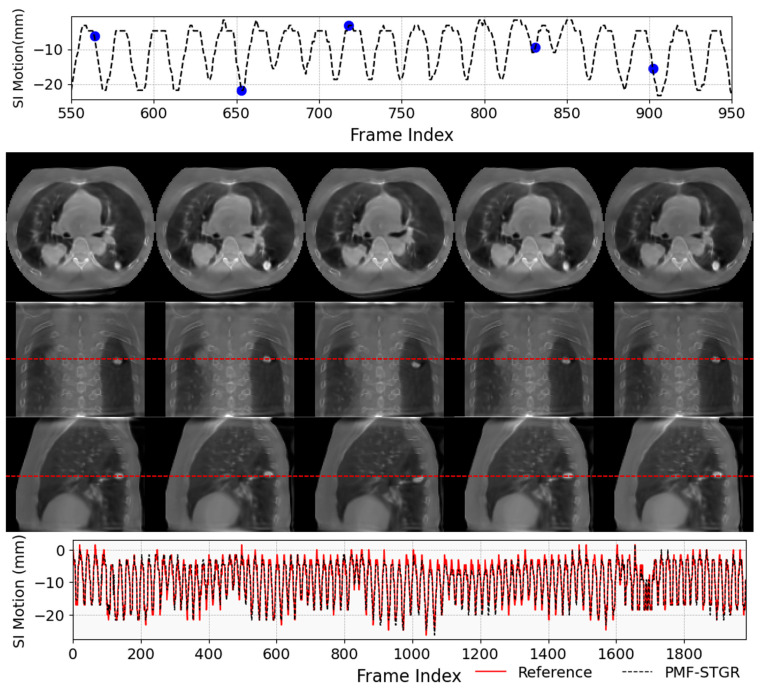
PMF-STGR reconstructed dynamic CBCTs. Row 1: superior-inferior (SI) motion trace; blue markers indicate the motion states visualized. Rows 2–4: reconstructed CBCT volumes at the selected states. Row 5: SI motion trajectory estimated by PMF-STGR (solid) versus a reference trajectory extracted with the Amsterdam–Shroud (AS) projection method (dashed). Figure is reproduced from [Xie, J. et al. [128] Time-resolved dynamic CBCT reconstruction using prior-model-free spatiotemporal Gaussian representation (PMF-STGR), Phys. Med. Biol., 2025] under CC BY 4.0.
